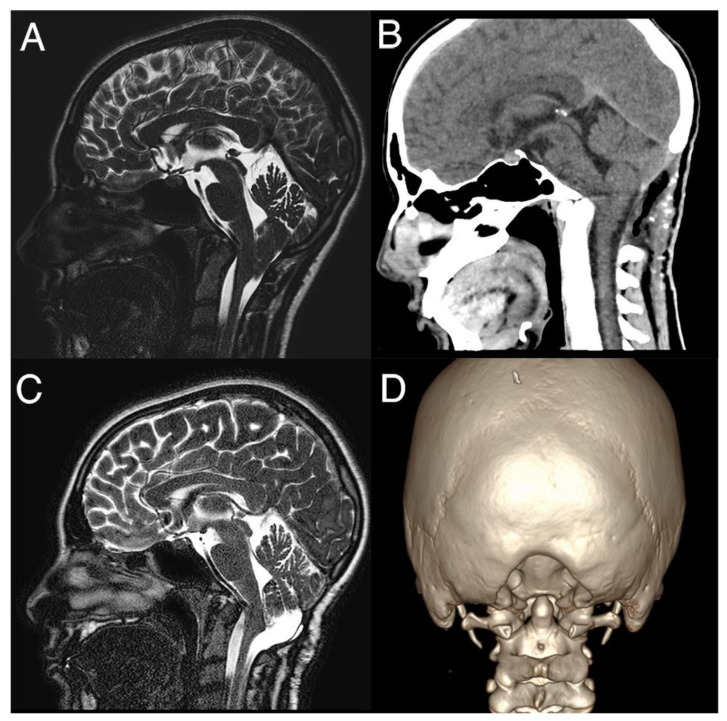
Chiari 1.5 malformation: the preoperative T2-weighted mid-sagittal scan demonstrates the displacement of the cerebellar tonsils and hindbrain below the foramen magnum (A). The patient was submitted to PFDD: the postoperative CT scan (B) with 3D reconstruction (coronal view in (D)) showed the entity of bony decompression, with CSF flow in the posterior fossa and reduction of tonsil descent (C).
